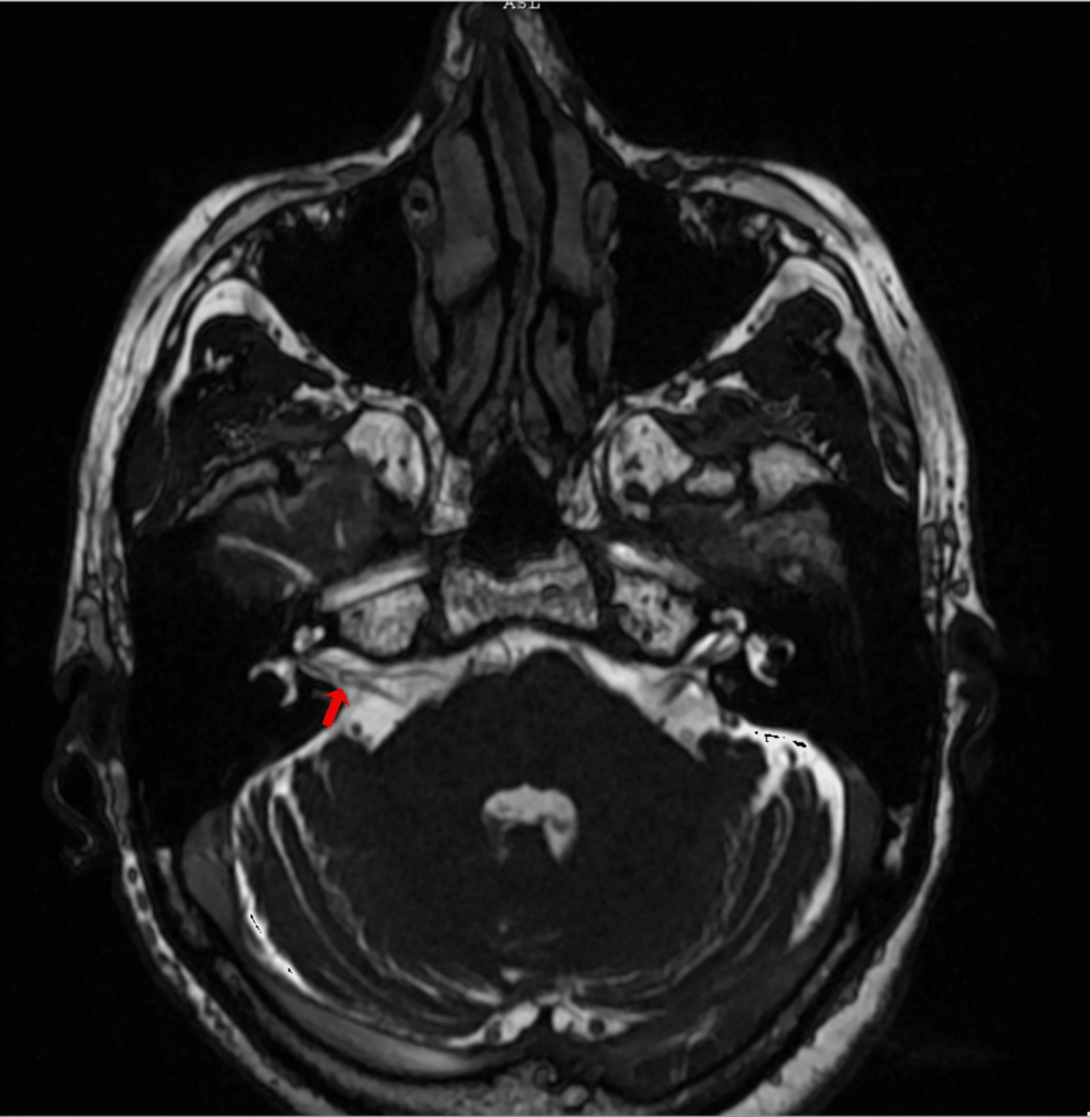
Axial FIESTA brain MRI of patient no.2 Red arrow: Right-sided neurovascular compression (Chavda classification grade II) FIESTA: Fast imaging employing steady-state acquisition
